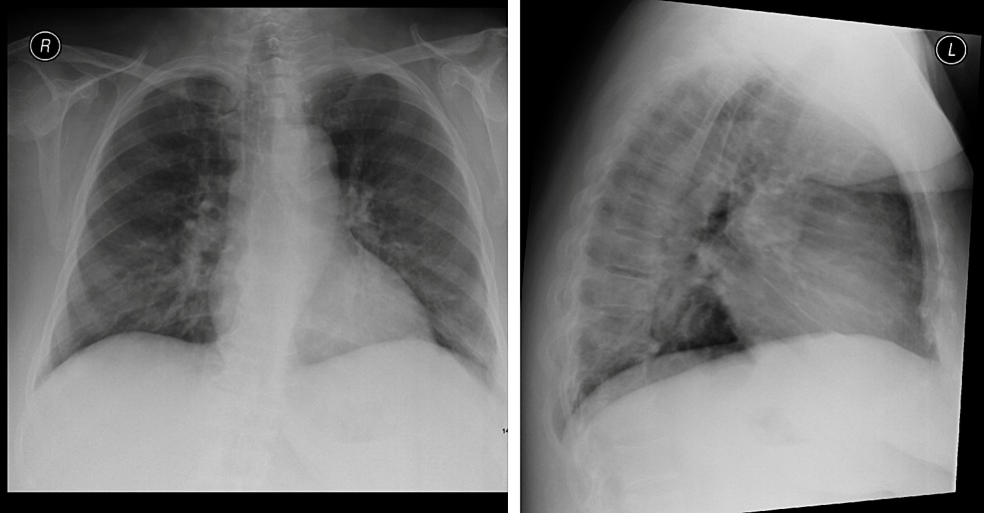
Chest x-ray showing bilateral improvement in the patient's infiltration, which was associated with clinical improvement
